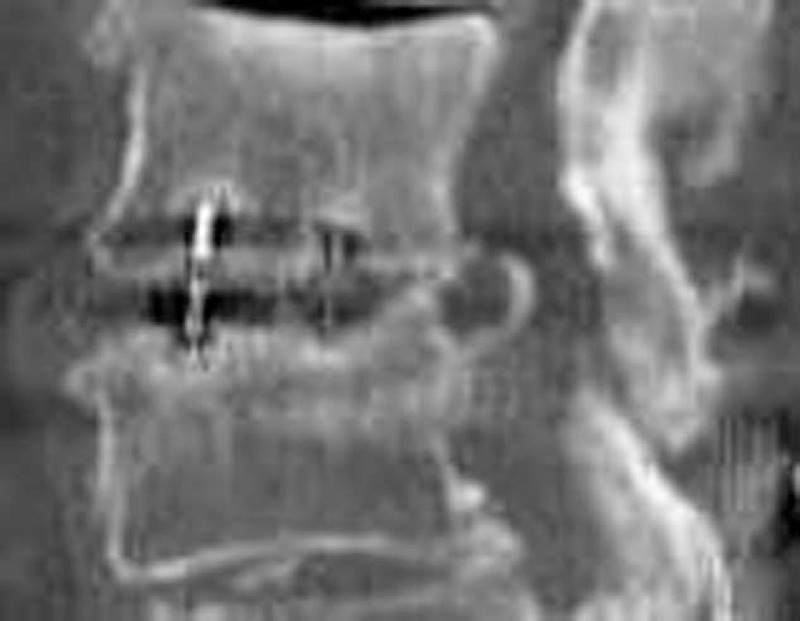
After 3 month pain-free interval, neurogenic claudication symptoms returned. Six-month postoperative CT scan shows subsidence of graft and ossification of annular bulge causing stenosis.
